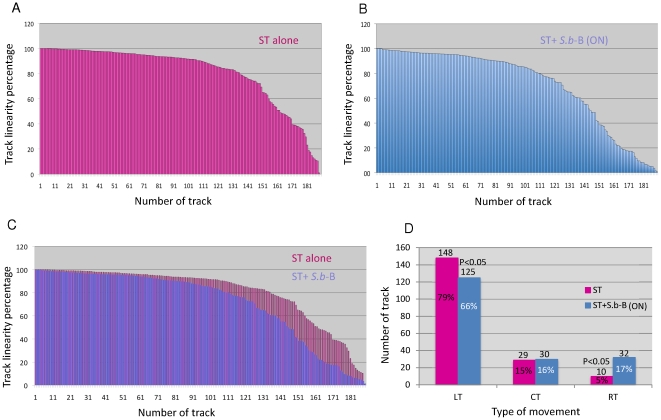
Comparison between the track linearity (LT) of ST incubated alone with T84 (A) or cells incubated overnight (ON) with *S.b*-B (*S.b*-B(ON)) and exposed to ST (B). Tracks are presented in descending order implying that track number in “x” axis did not correspond to the track number attributed by Image-J. 187 ST- alone tracks are presented in panel A and 187 tracks of bacteria in the presence of *S. b*-B(ON) are presented in panel B. In panel C are represented the superposition of the all tracts from panel A and B. Quantification of different types of tracks of ST alone or ST in the presence of *S.b*-B are presented in panel D. The percentages marked in each column correspond to: the number of specific track type/the total number of tracks (187) for each condition ×100. The data derived from a sequence using MTrack J processing software. LT: linear trajectories; CT: curvilinear trajectories, RT: rotatory trajectories.
